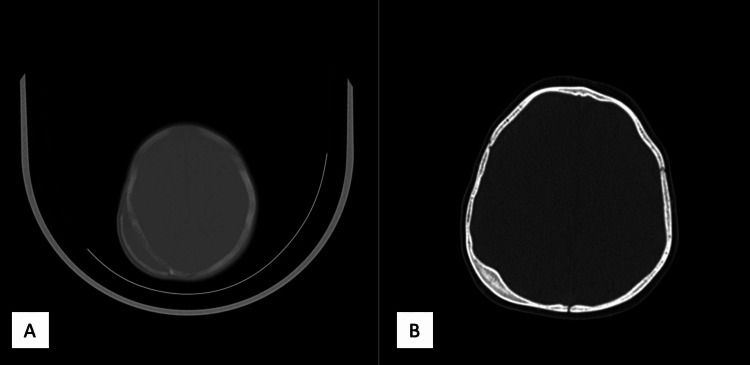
(A) An ossified cephalohematoma was seen in the right parietal region in a two-month-old baby. (B) At the 12th month, the ossified hematoma is seen to be resorbed in the control CT scan, but cranium thickening is observed in the area where the cephalohematoma is present.
